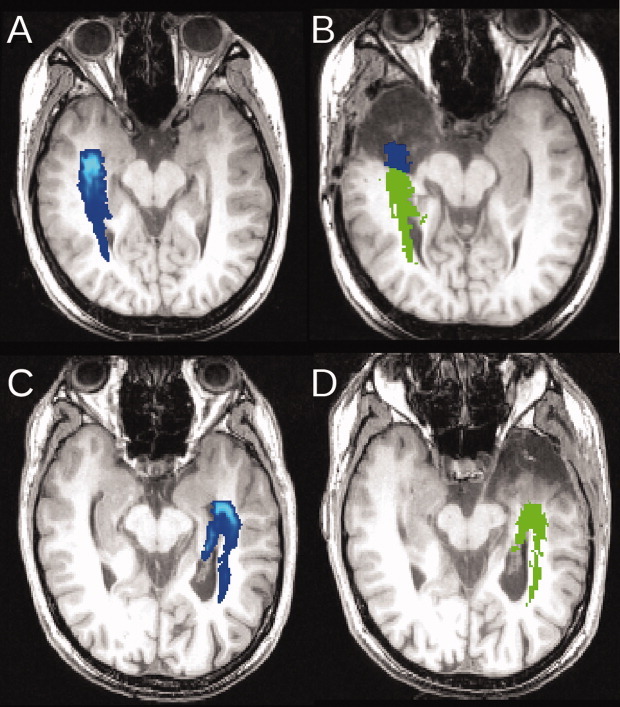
Preoperative structural T1-weighted image and optic radiation (A) and postoperative structural T1-weighted image with propagated preoperative tractography (B) show that part of the optic radiation was resected (blue) in patient 7, who developed a severe visual field deficit (VFD). Corresponding preoperative (C) and postoperative images (D) are shown in patient 12, who did not develop a VFD.
